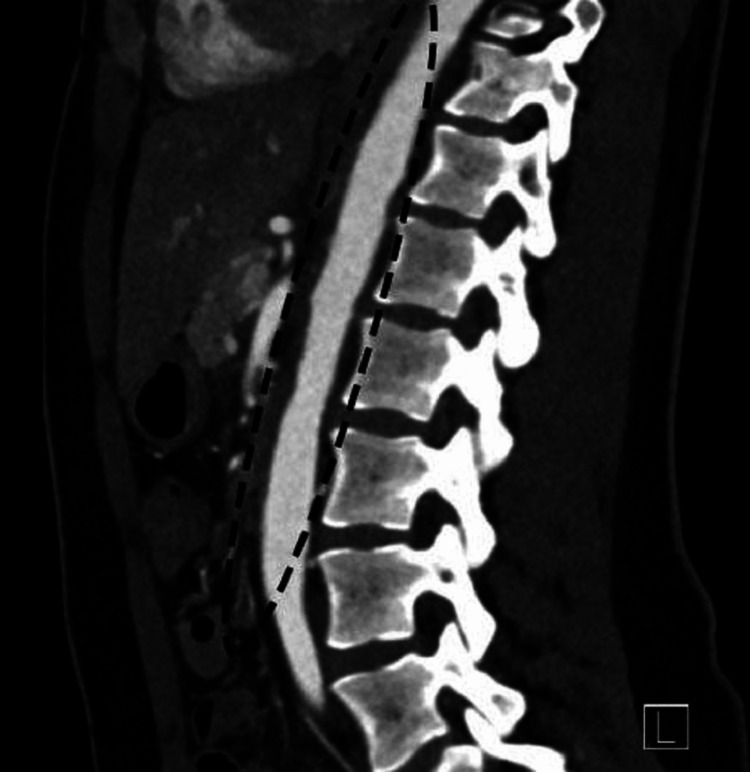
Sagittal CT angiogram of the abdomen showing an undulating appearance of the descending and abdominal aorta (black dashed ellipse). CT: computed tomography
